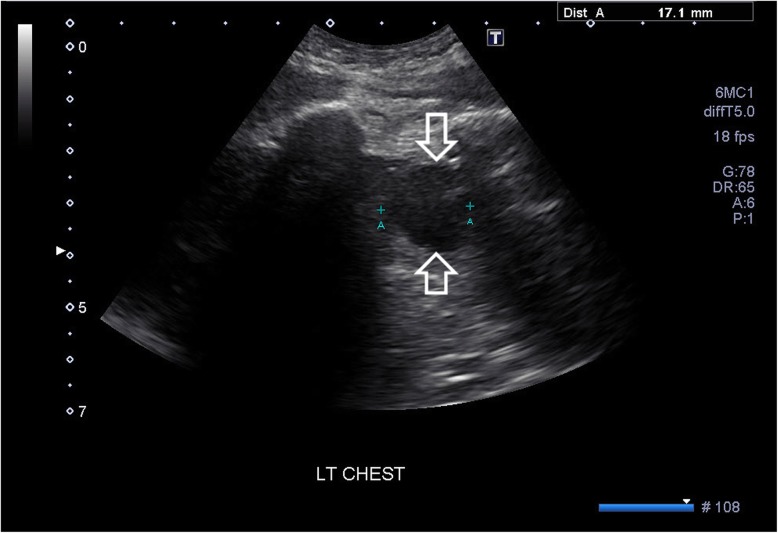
Grey scale ultrasound image of the left intercostal artery pseudoaneurysm. Arrows delineate the pseudoaneurysm
